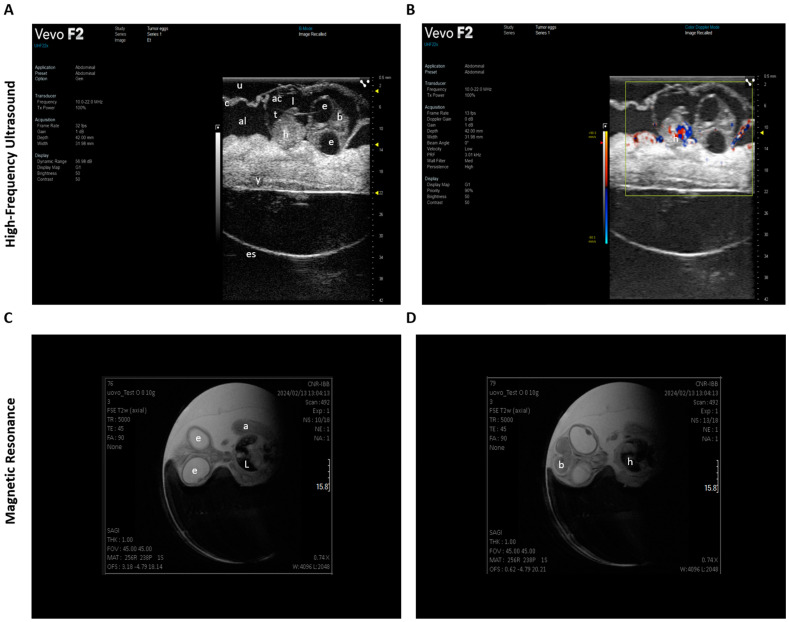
Multimodal imaging of CE. (A) B-mode and (B) color doppler mode transversal section at ED7, imaged with UHF 10–22 MHz transducer (acquisition parameters: frame rate 32 fps, depth 42.00 mm, width 31.98 mm, transmitted power 100%, dynamic range 56.98 dB) [Visual sonics Vevo F2 Ultrasound scanner]. (C,D) T2-weighted sequence to assess the organogenesis at ED13; the high soft tissue contrast of the MRI allows for identifying the different organs during the development of the CE [7T MR Solutions]. e: eye, a: abdomen, L: liver, b: brain, h: heart, t: thorax, l: limb, c: CAM, y: yolk, al: albumen, ac: amniotic cavity, u: ultrasound coupling gel, es: egg shell.
